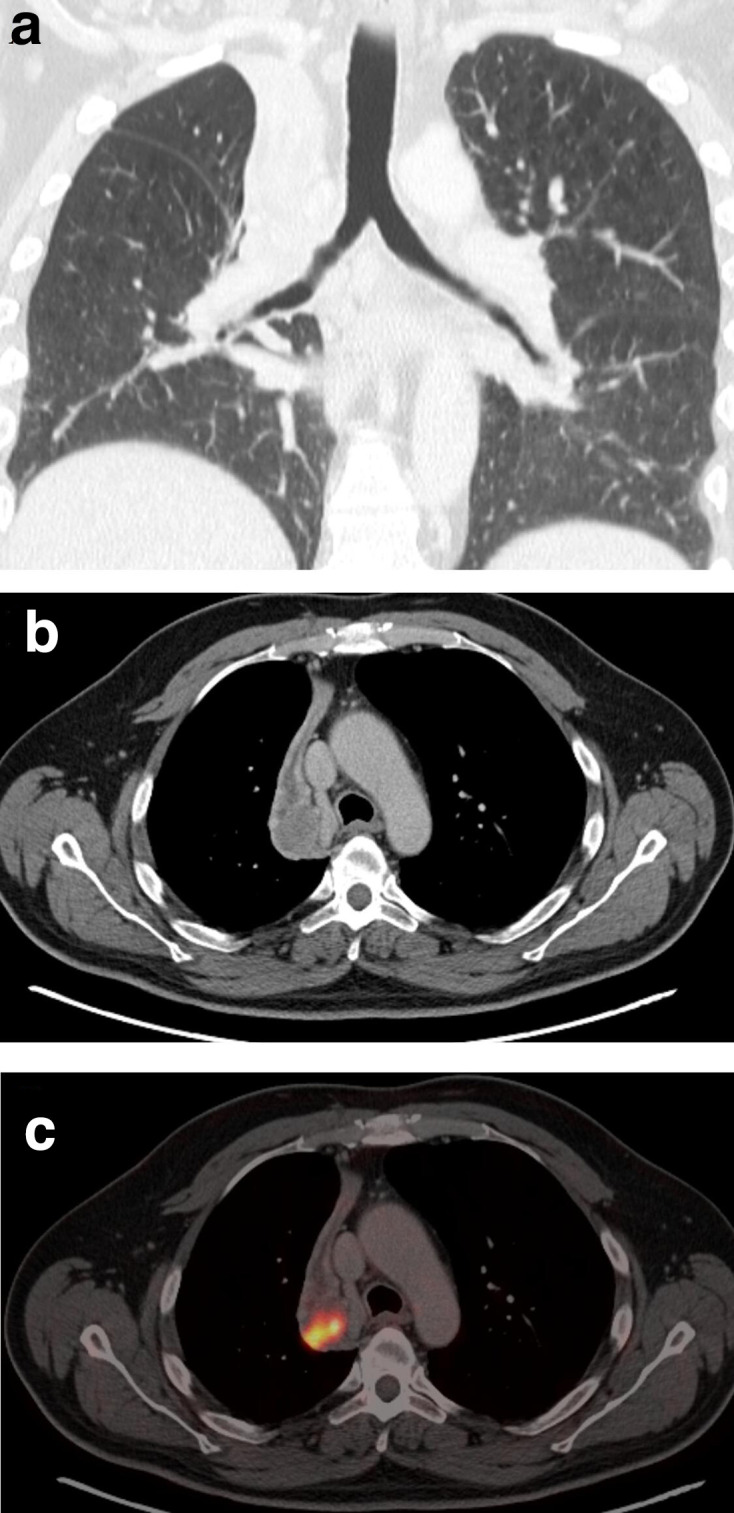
(a) A 65-year-old patient with NSCLC and right upper lobe atelectasis. On the contrast-enhanced CT (b) an inhomogeneous mass is seen at the level of the right hilum that obliterates the right upper lobe bronchus. The exact tumour diameter cannot be assessed accurately. (c) FDG -PET/CT shows focally increased FDG uptake in the tumour, while the post-stenotic atelectatic lung shows only a moderate FDG uptake. In this patient, the metabolic information helps to correctly assess tumour dimension. FDG, fludeoxyglucose; NSCLC,non-small cell lung cancer; PET, positron emission tomography
